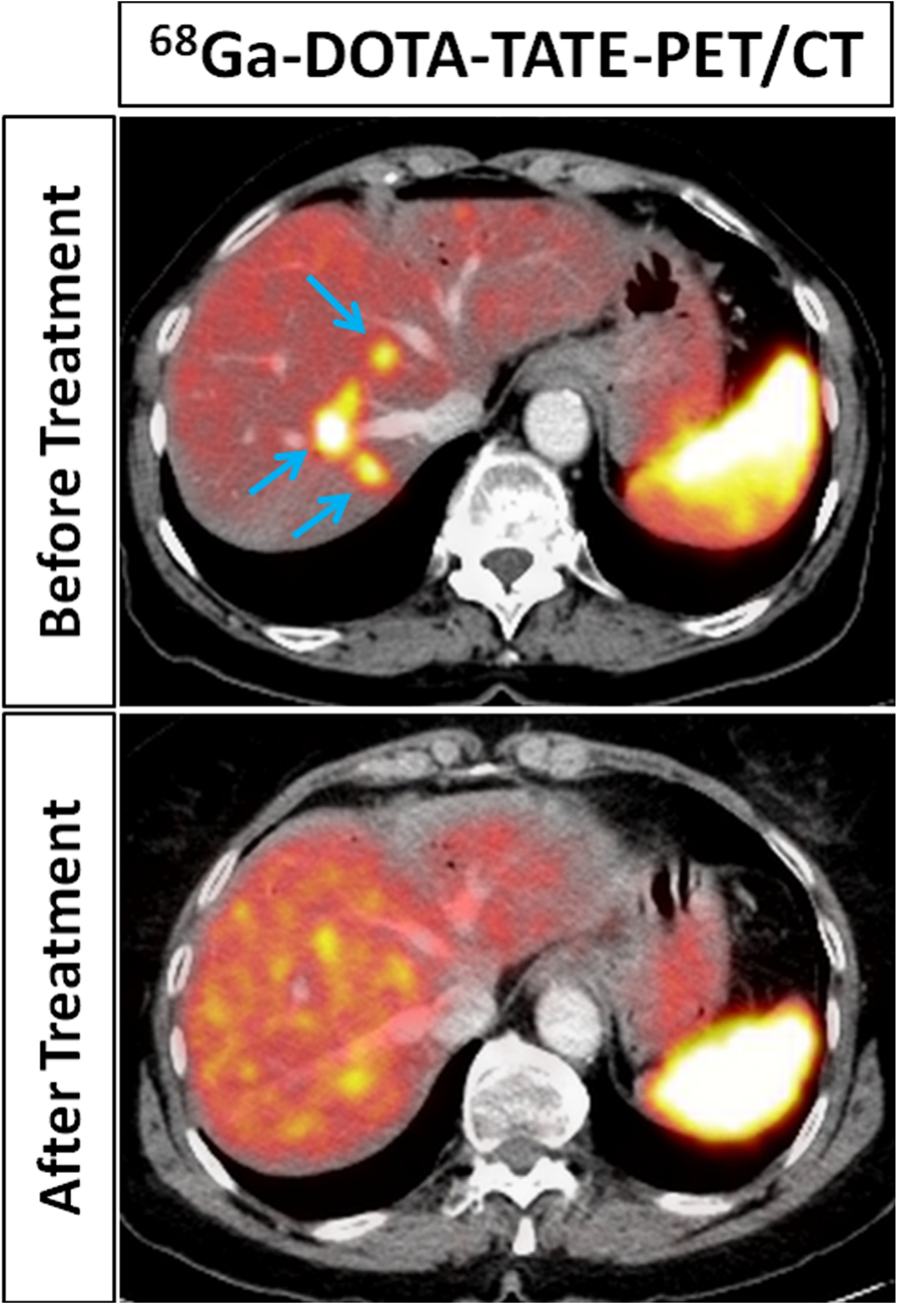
^68^Ga-DOTA-TATE-PET/CTs in a patient with metastatic neuroendocrine tumor before (top row) and after (bottom row) radionuclide therapy with ^177^Lu-DOTA-TATE. The pre-treatment diagnostic scan showed multiple avid liver metastases (arrows), implying that they would also bind the ^177^Lu-labelled therapeutic ‘sister agent’, therefore predicting the treatment response that was ultimately demonstrated on the post-therapeutic PET/CT. Physiologic splenic tracer uptake is seen on both studies
